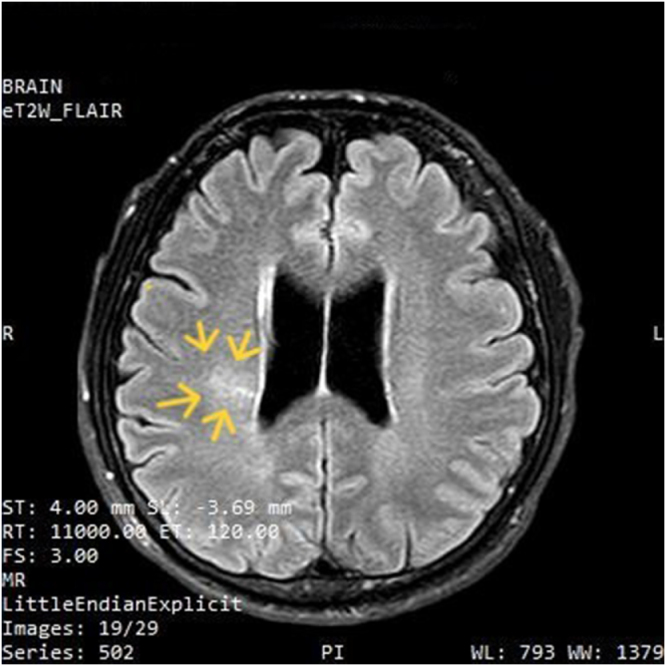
FLAIR image. Arrow heads showing focal area in the corona radiata of the right frontoparietal lobe that demonstrates band of high signal intensity suggestive of focal cerebral edema.
